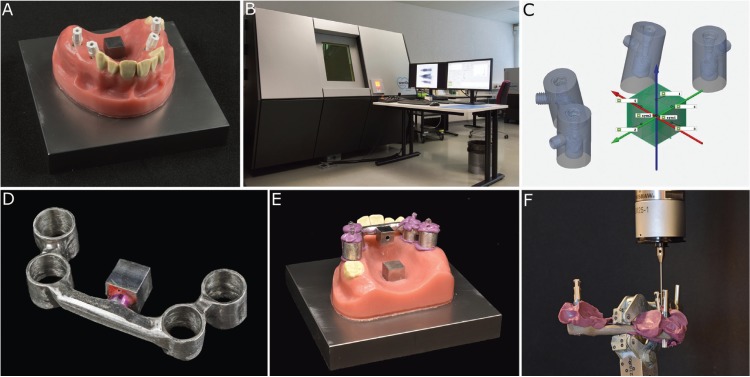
A: Implant master model (IMM) with tightened scanbodies on the premolar (15° inclination) and molar (0° inclination) implants and the reference cube in the middle of the palate. B: Multisensor coordinate measuring machine using X-ray tomography (TomoScope S). C: Reference file (patient equivalent) with the implant-abutment interface centers (IAICs) from the computed tomography (green points). D: Custom made measuring aid (CMA) with the inherent reference cube. E: CMA coded on the IMM. F: Measurement of the transferred CMA with tightened laboratory analogues using the coordinate measuring machine (CMM).
